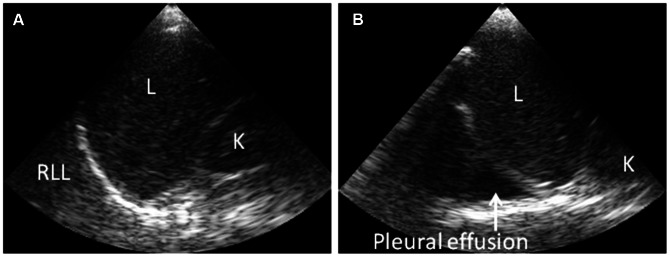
Ultrasonography findings showing presence or absence of pleural effusion in dengue patients. Handheld ultrasonography at the bedside with patient in supine position during examination. (A) Normal right costophrenic angle. (B) Pleural effusion in the right costophrenic angle (arrow). Abbreviations: L: liver; K: kidney, RLL: right lower lobe of lung.
